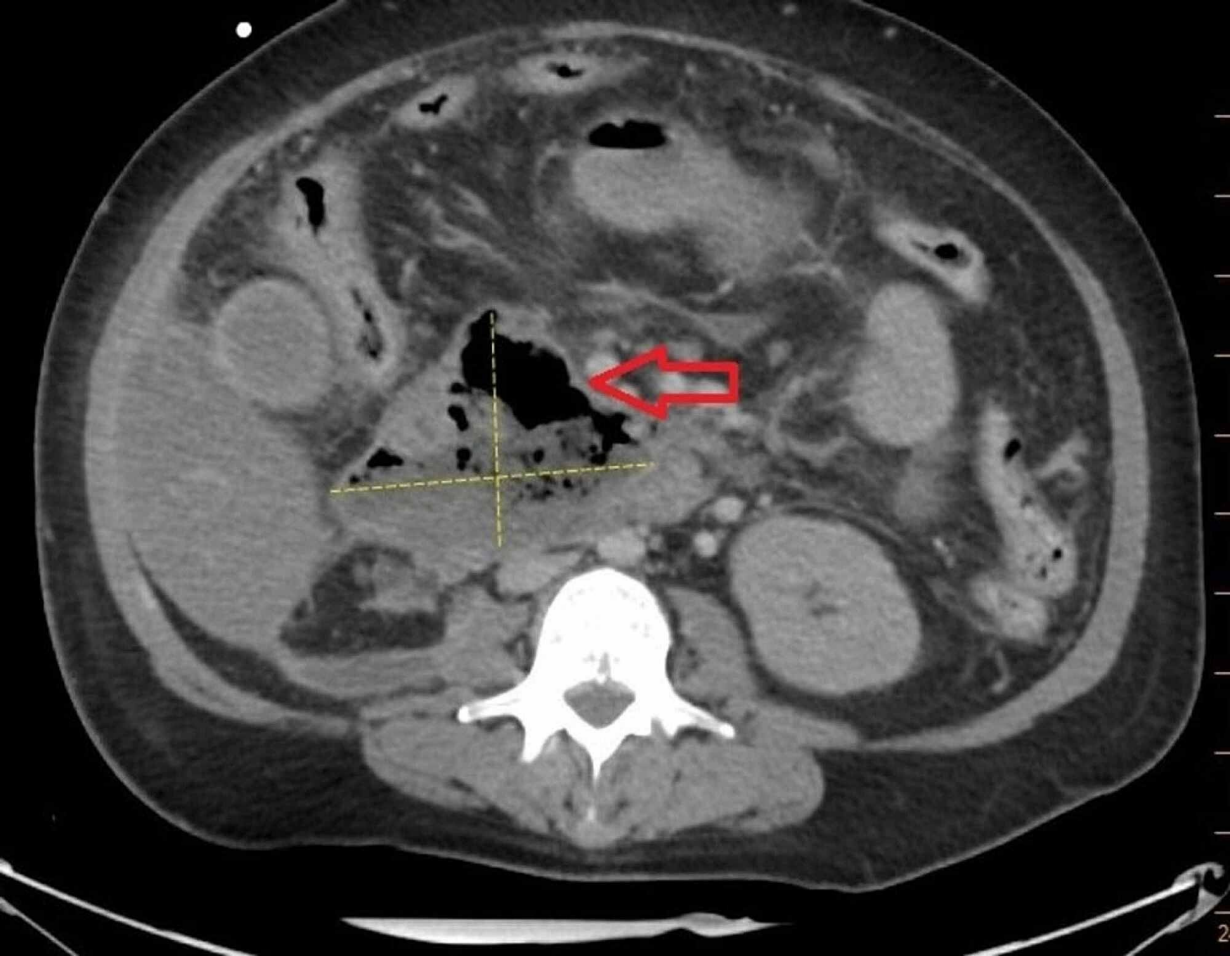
CT scan of abdomen showing pancreatic abscess
